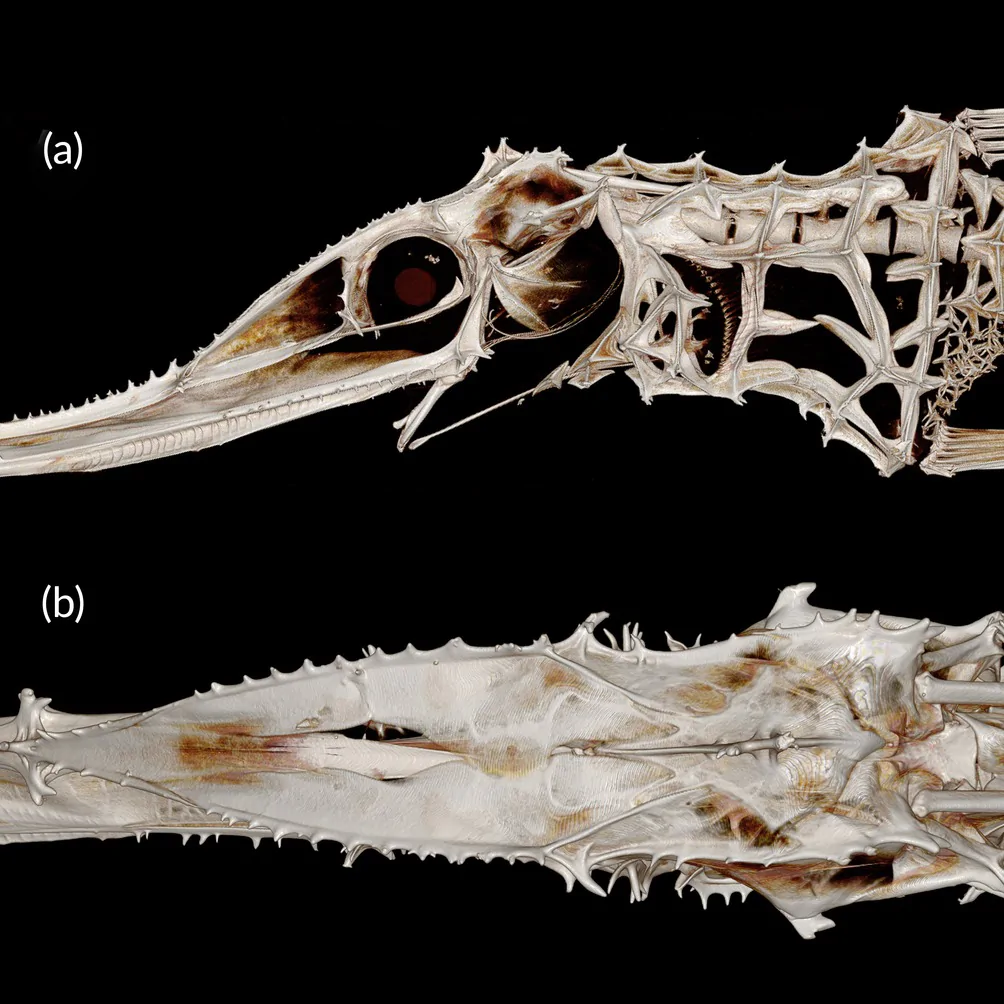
Micro‐CT reconstruction of the supraoccipital crest of *Solenostomus paegnius*, female, CAS 78331: (a) lateral view; (b) dorsal view.
